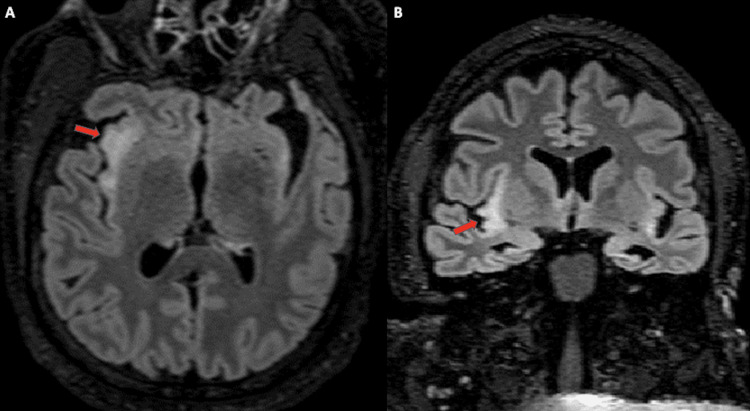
Axial (A) and coronal (B) T2 FLAIR MRI obtained in 2022 Demonstrates persistent hyperintensity of the right insula and new severe atrophy of the temporal lobes/hippocampi (arrows).
